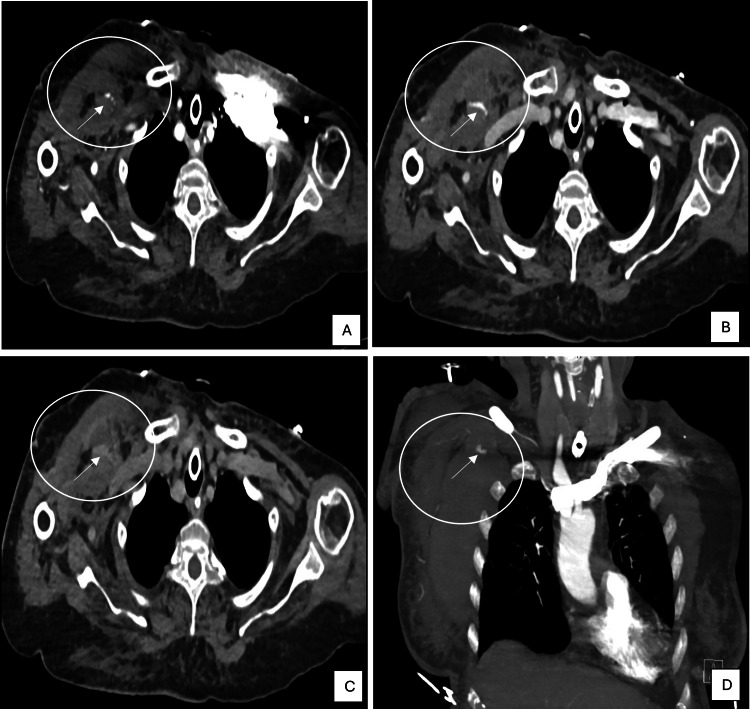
Computed tomography: axial arterial phase (A), axial venous phase (B), axial delayed phase (C), and MIP coronal arterial phase (D) Computed tomography showing the focus of active arterial extravasation progressing on the venous and delayed phases in the vicinity of the right axillary artery (arrows) with large right muscular pectoral/subpectoral hematoma (white circle) MIP: maximum intensity projection
